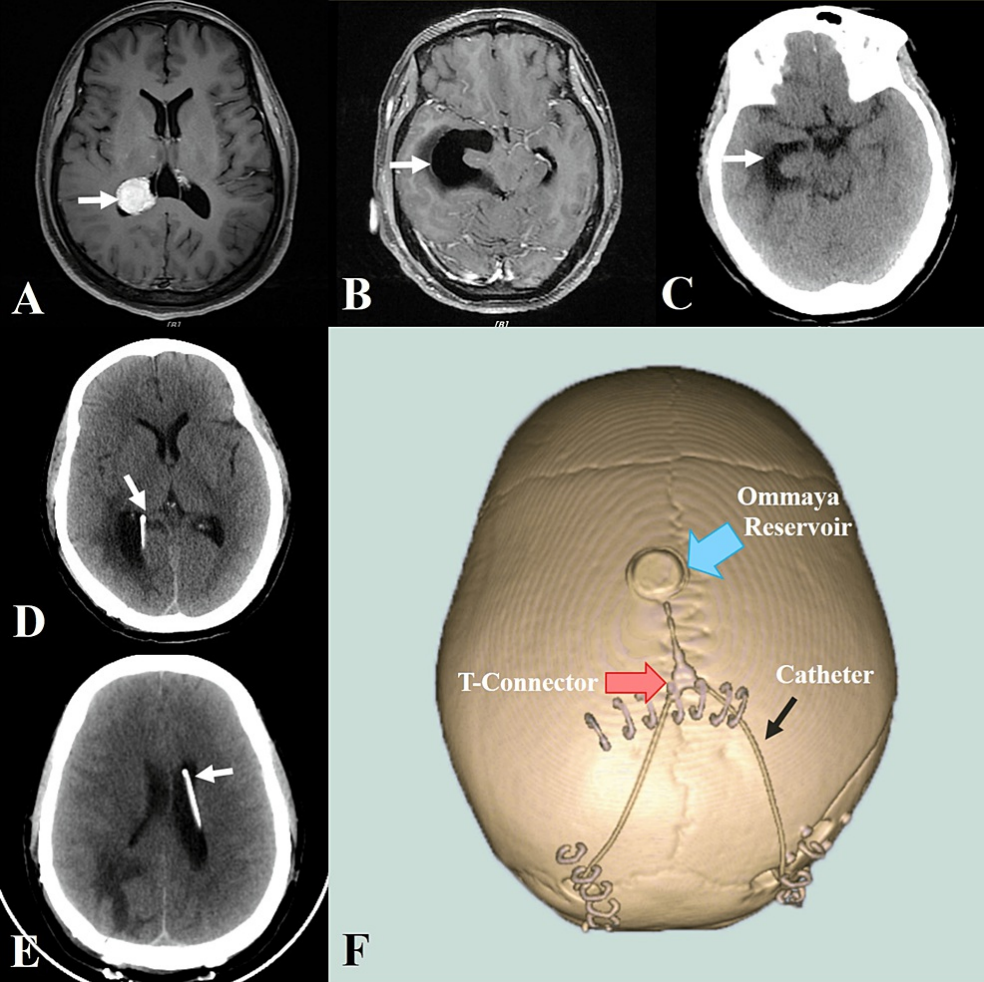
Image of case 2 A: MRI showed a meningioma in the right trigone, measuring 2.1 cm × 2.2 cm. B: MRI demonstrated a right temporal horn width of 3.46 cm on one month after surgery. C: CT showed a right temporal horn width of 2.72 cm on the first day after the intraventricular shunt. D: CT showed the catheter tip on the right trigone. E: CT showed the catheter tip on the left frontal horn. F: 3D reconstruction showed the position of the T-connector, Ommaya reservoir, and extracranial catheter.
